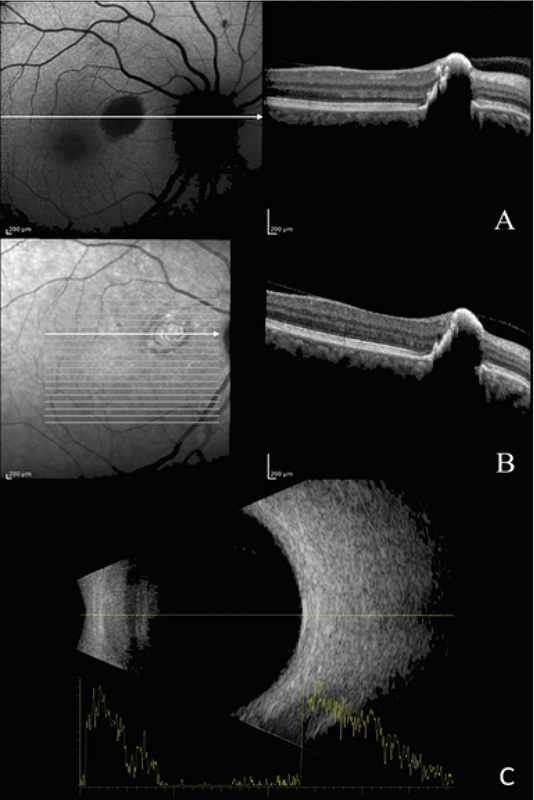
Optical coherence tomography (OCT) showing a retinal lesion with high surface reflectivity, deep optical shadowing, and full-thickness retinal involvement. Image (A) was taken in 2009, and image (B) in 2017. The arrows on the left side autofluorescence (AF) and infrared images show the level of B scans that although not registered pass through the same location based on fundus landmarks. The lesion shows hypoautofluorescence in AF imaging. (C) B scan ultrasonography reveals a flat lesion with high internal reflectivity.
